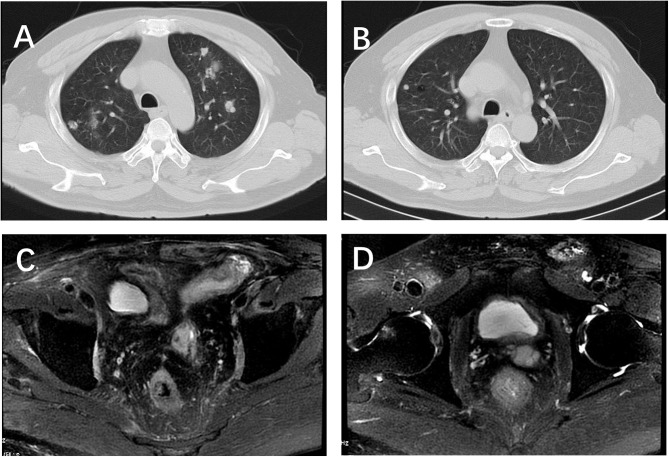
Chest CT and pelvic MRI after 5 circles etoposide chemotherapy. (A,B) 80% decrease in size of measurable lung nodules; (C,D) no visible recurrence shown on the pelvic MRI.
